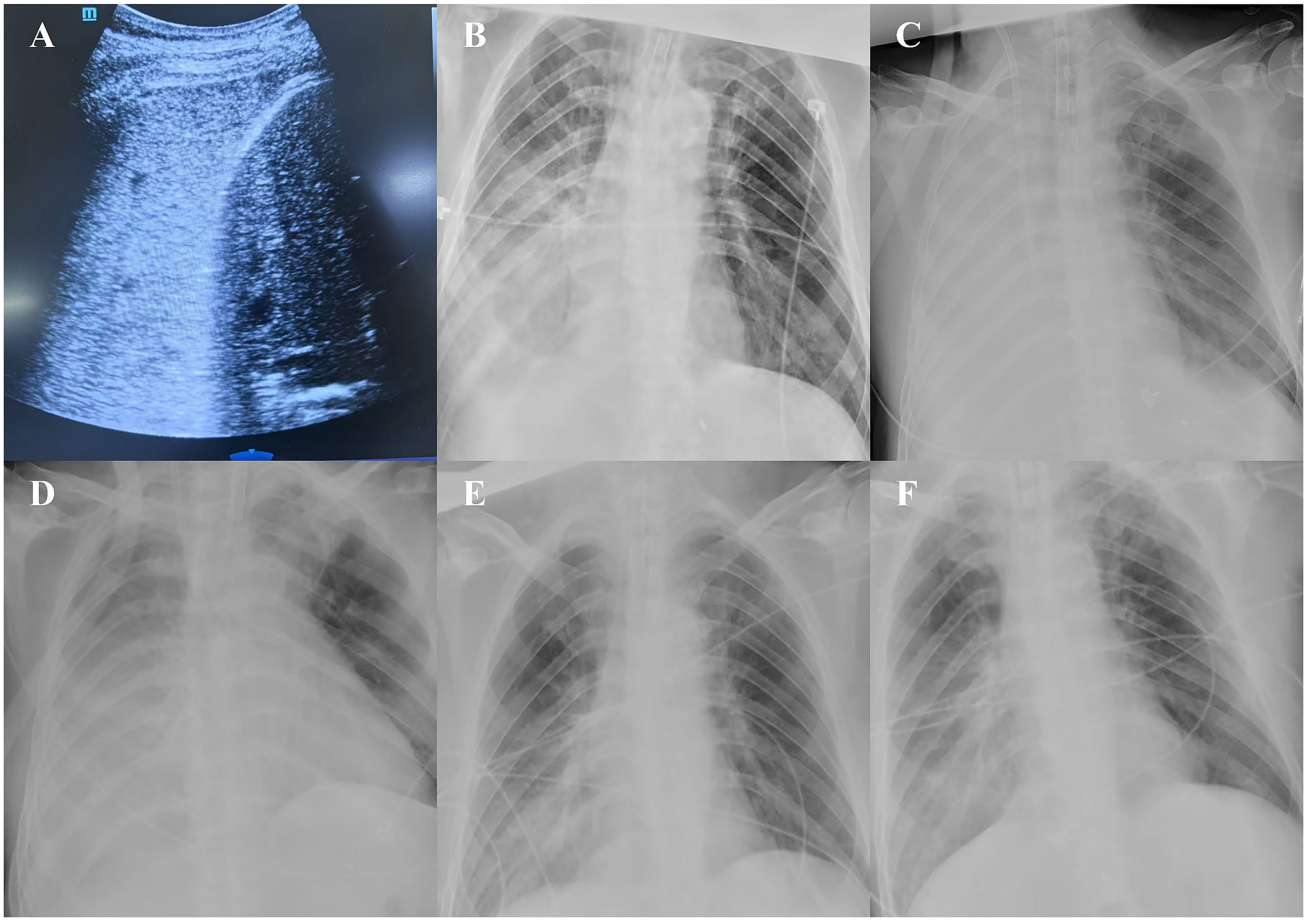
Ultrasonography and chest radiography. (A) Chest ultrasonography shows “hepatic-like consolidation” in the right lower lung. (B) Chest radiograph corresponding to the ultrasonographic findings (before ECMO support and fluid resuscitation). (C) Chest radiograph after fluid resuscitation with ECMO support. (D–F) Chest radiographs during the resolution phase.
